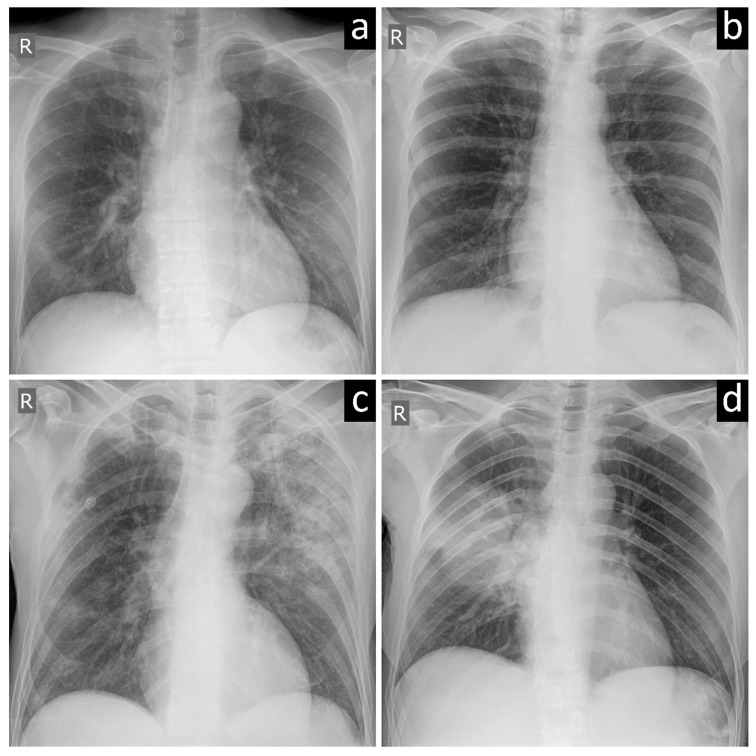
Chest X-ray images of subjects with (a) COVID-19 pneumonia, (b) negative examination, (c) viral pneumonia, and (d) bacterial pneumonia.
